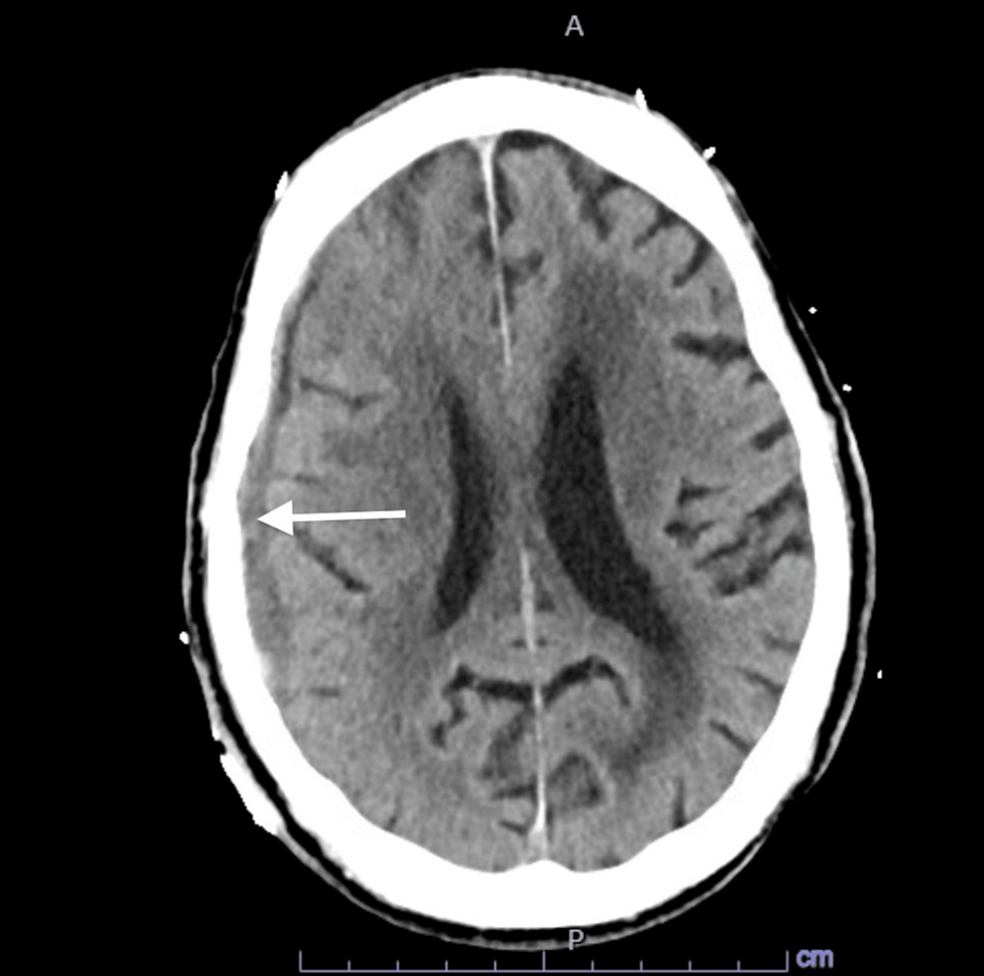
CT scan of the head Computed tomography (CT) scan of the head shows a relatively stable size of the right-sided subdural hemorrhage (arrow)
